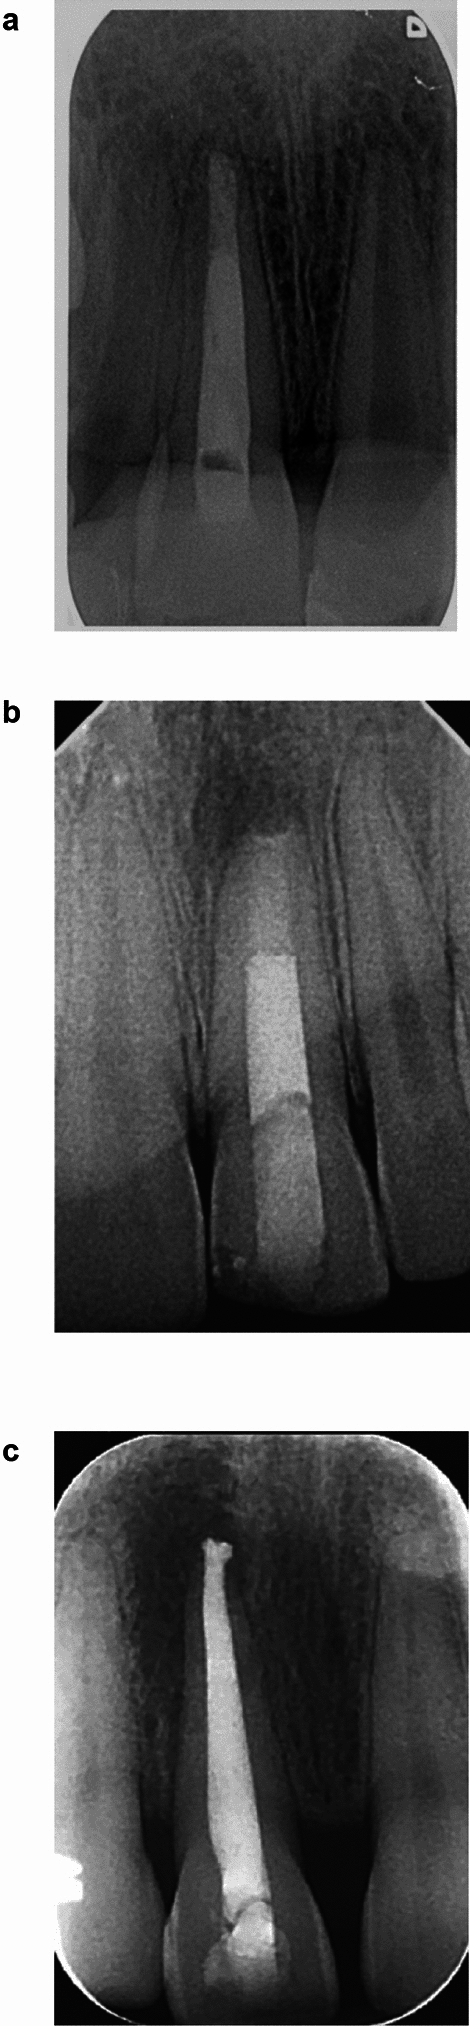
a Periapical radiograph demonstrating the desired technical quality of an MTA apical plug and obturation on a maxillary right central incisor (UR1). b Periapical radiograph demonstrating the desired technical quality of a Biodentine apical plug and obturation on a maxillary left central incisor (UL1). c Periapical radiograph demonstrating the desired technical quality of a TotalFill Putty apical plug and obturation on a maxillary right central incisor (UR1)
